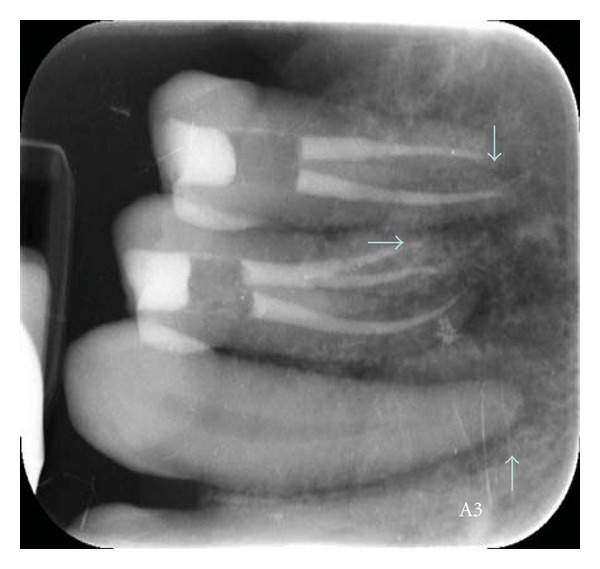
Case 5. Periapical radiograph.
